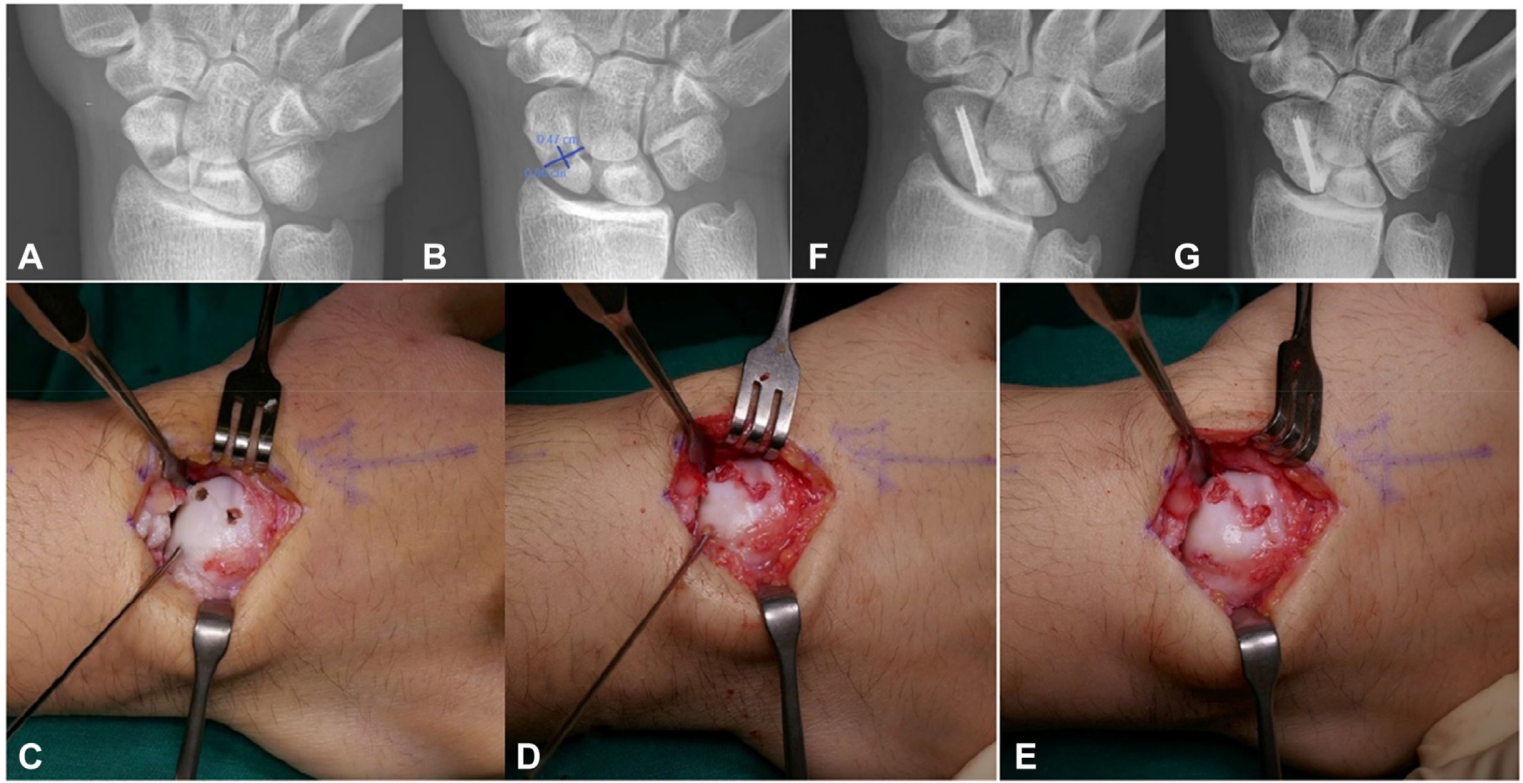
Case no 7. Scaphoid fracture bone grafting “Perforate and Fill” technique. (A) Radiograph showing proximal pole scaphoid fracture with radiolucent ellipsoidal cavitation from bone resorption. (B) Showing the size of cavitation measuring L = 9.4 mm and H = 4.7 mm, with an area of 139 sq mm and estimated volume of 1.7 mL. (C) Showing good cartilage cohesion of a fibrous nonunion, placement of the headless compression screw guide wire, and access to the fracture cavity with two 4 mm burr holes. (D) Morselized bone grafts were filled into one of the perforations in small batches, and further compacted in with a small bone tamp. (E) A predetermined headless compression screw of the chosen size and length is inserted through the guide wire till well seated deep to the cartilage. (F) Postoperative radiograph at 76 days showing a well filled cavitation and union of the fracture. (G) Radiograph 17 months postfixation showing a well united fracture of the scaphoid.
